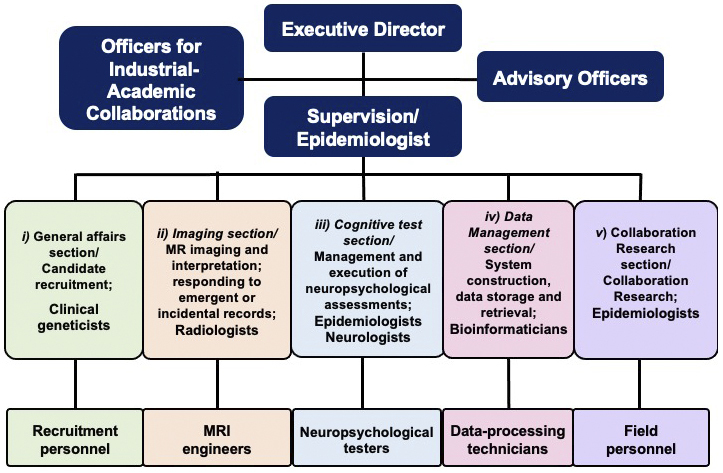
Organization, leadership, and staffing of the TMM Brain MRI Study. Experts from a variety of disciplines bring together their academic knowledge and experience to manage the TMM Brain MRI Study through five special teams. Experts include clinical geneticists, radiologists, epidemiologists, bioinformaticians, and neurologists.
